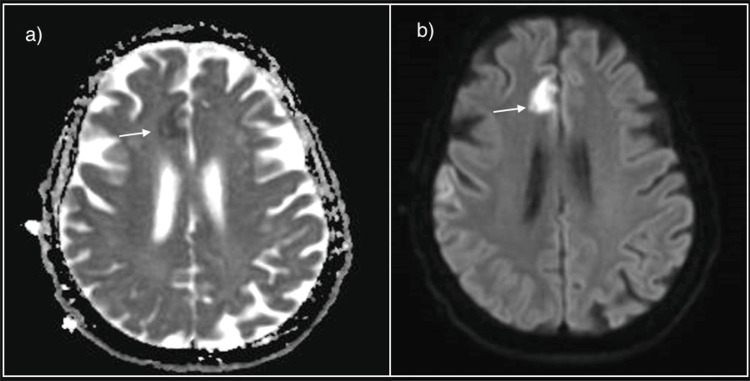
Magnetic resonance imaging of the brain a) low signal on the Apparent Diffusion Coefficient (ADC); b) restricted on the Diffusion-Weighted imaging in the right frontal lobe
